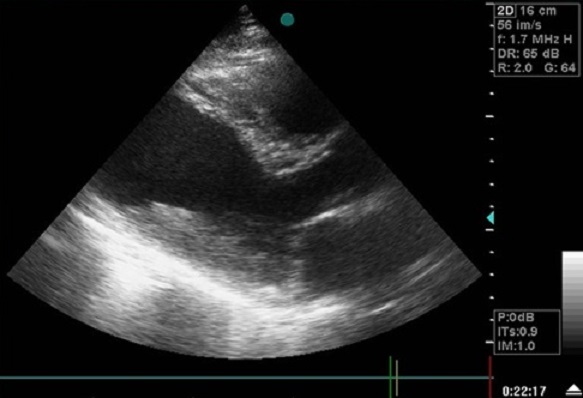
Coupe parasternal grand axe à l'ETT montrant un anévrysme ventriculaire gauche
